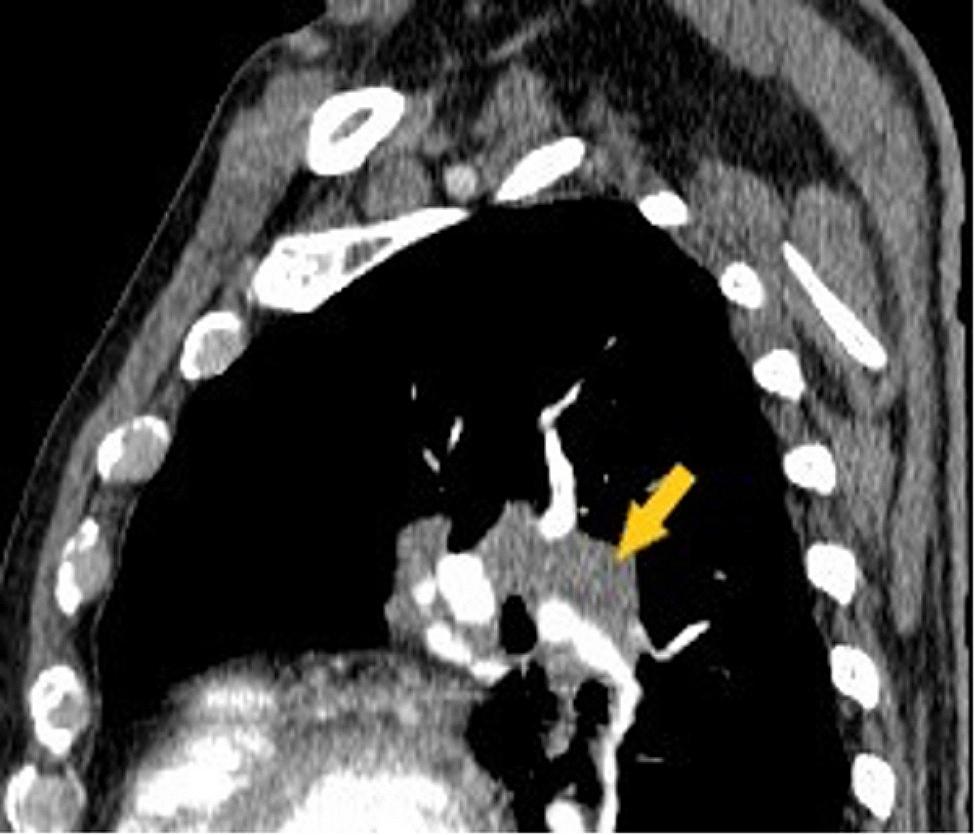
Chest CT examination, arterial time: lesional complex left hilum tumor mass-adenopathy (yellow arrow)
